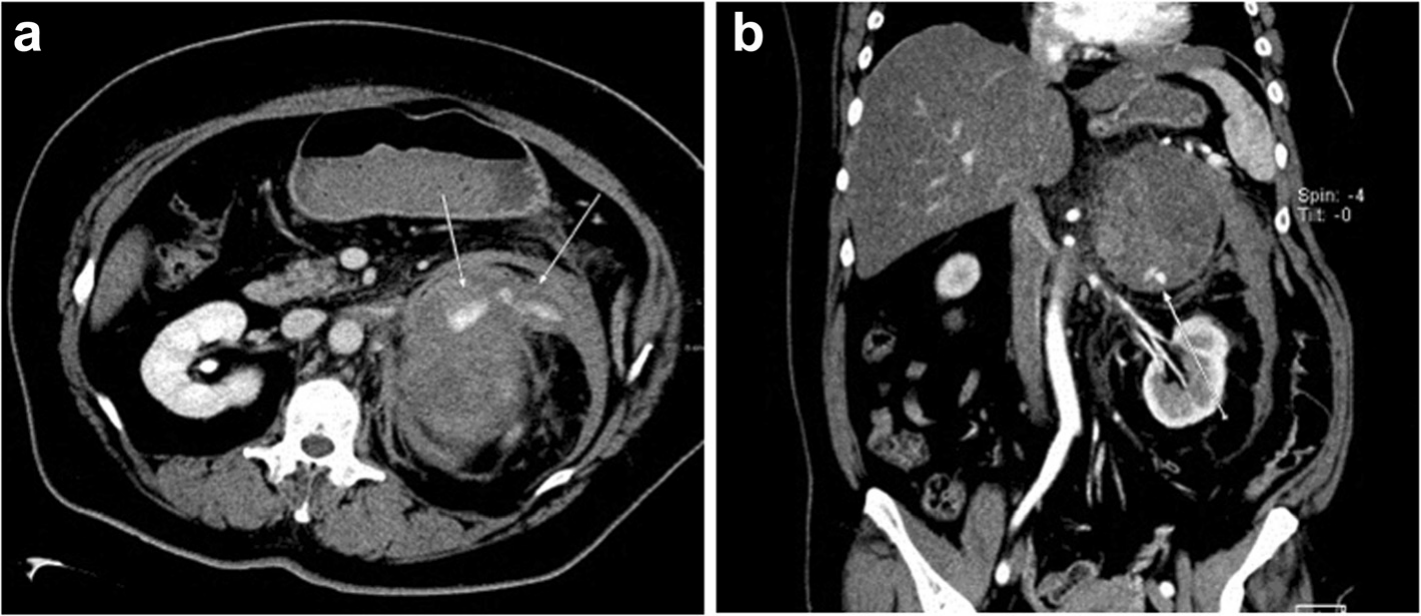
Contrast-enhanced CT findings. a. Emergency contrast-enhanced CT of the abdomen revealed a tumor-like soft mass accompanied by massive bleeding in the retroperitoneum (Transverse plane). b. Coronal plane.
